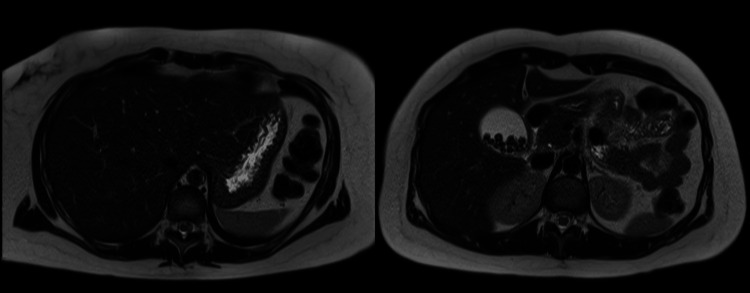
MRI: axial plane MRI: magnetic resonance imaging
